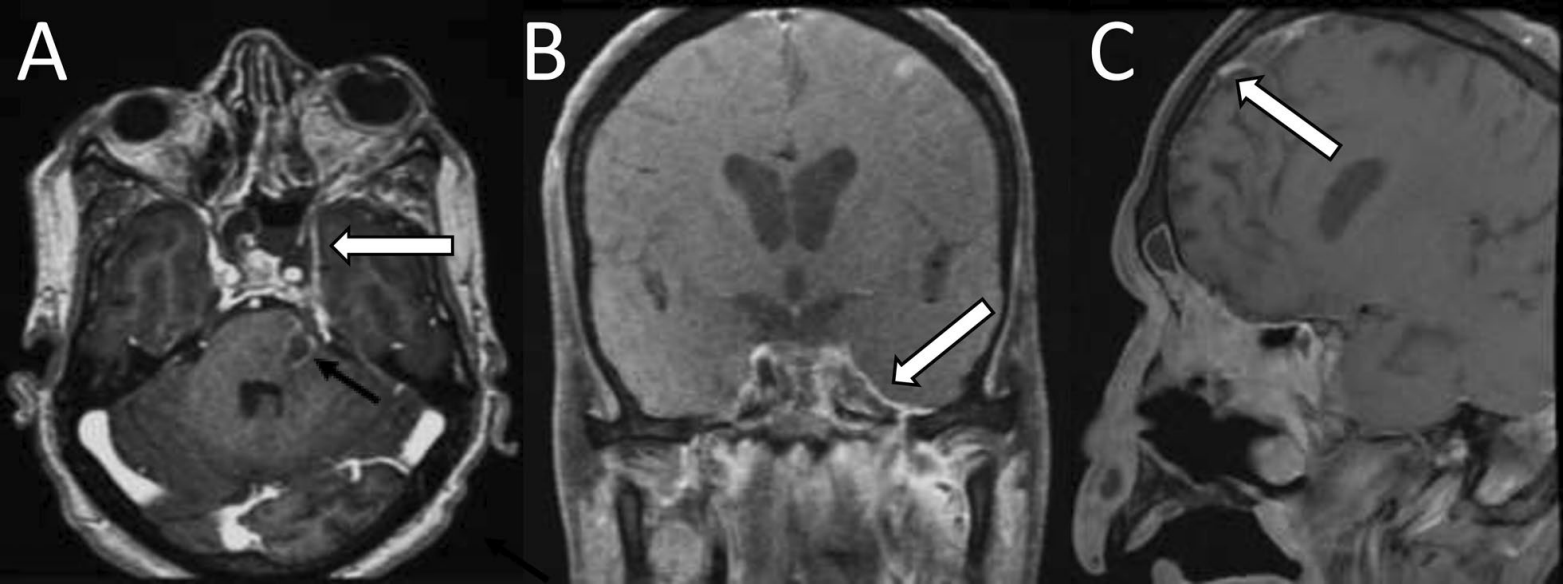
Pachymeningitis. Contrast-enhanced axial (A), coronal (B), and sagittal (C) T1-weighted fat-saturated images show pachymeningeal enhancement (white arrows) along the medial aspect of the left temporal and left frontal lobes. Image (A) also shows a ring-enhancing lesion in the pons suggesting a fungal abscess (black arrow)
